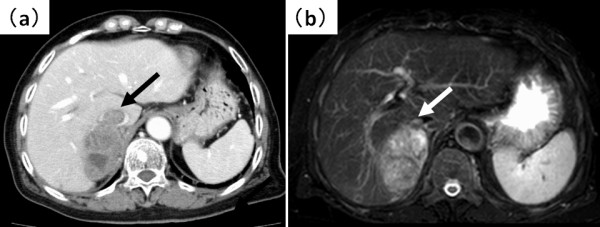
Abdominal computed tomography(CT)and magnetic resonance imaging. (a) Contrast enhanced CT and (b) fat-suppression T2-weighted images revealed a mass extending through the right adrenal vein into the inferior vena cava (IVC). Arrows designate the IVC tumor thrombus.
